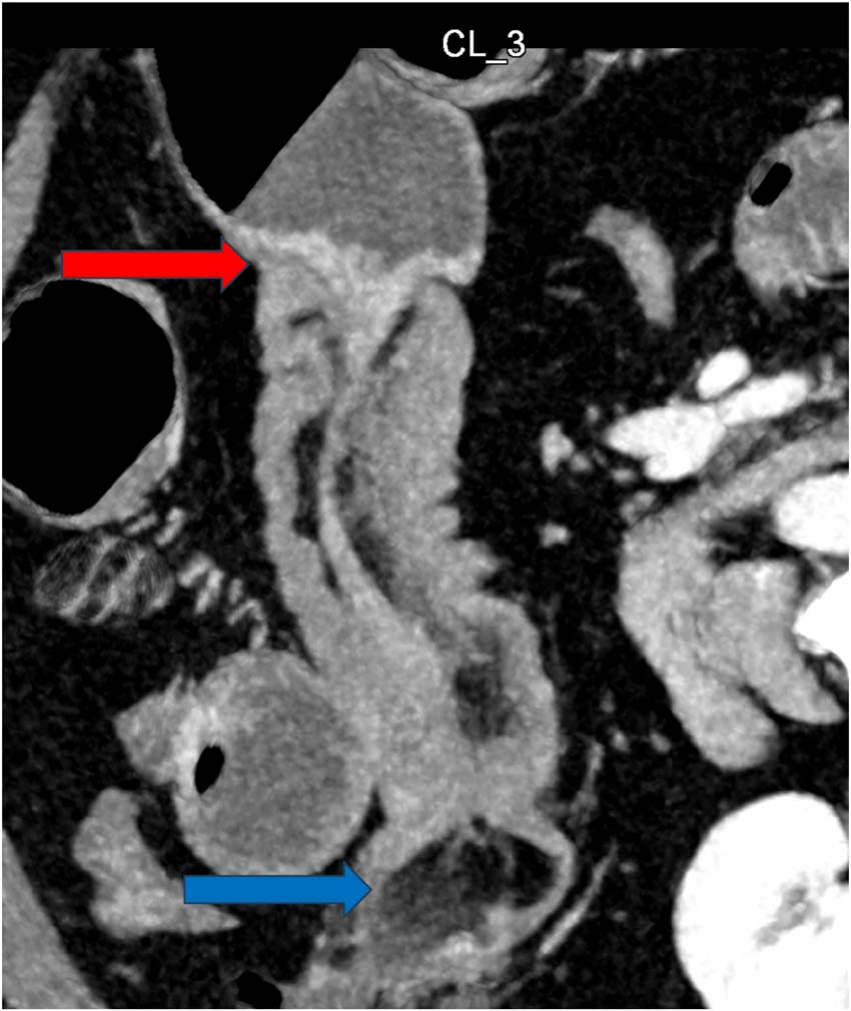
CT Abdomen: MPR reconstruction of the plane of intussusception showing a distal lipoma and proximal start of intussusception.
